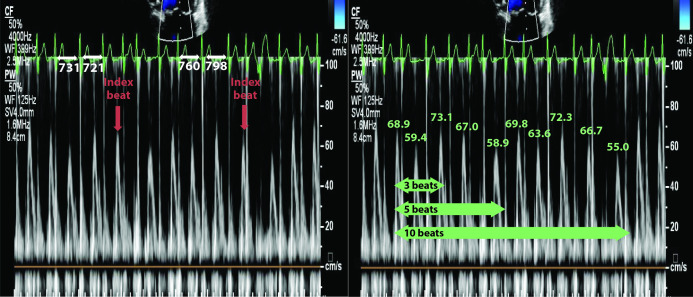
Index-beat approach versus conventional averaging of consecutive beats. Left panel: pulse wave mitral inflow Doppler using the index-beat method, with similar preceding and pre-preceding R-R intervals indicated by the red arrows. Values are the R-R interval length of the preceding cardiac cycle. Right panel: 3, 5 and 10 consecutive beats. Values are the peak E velocity measurement in cm/s.
